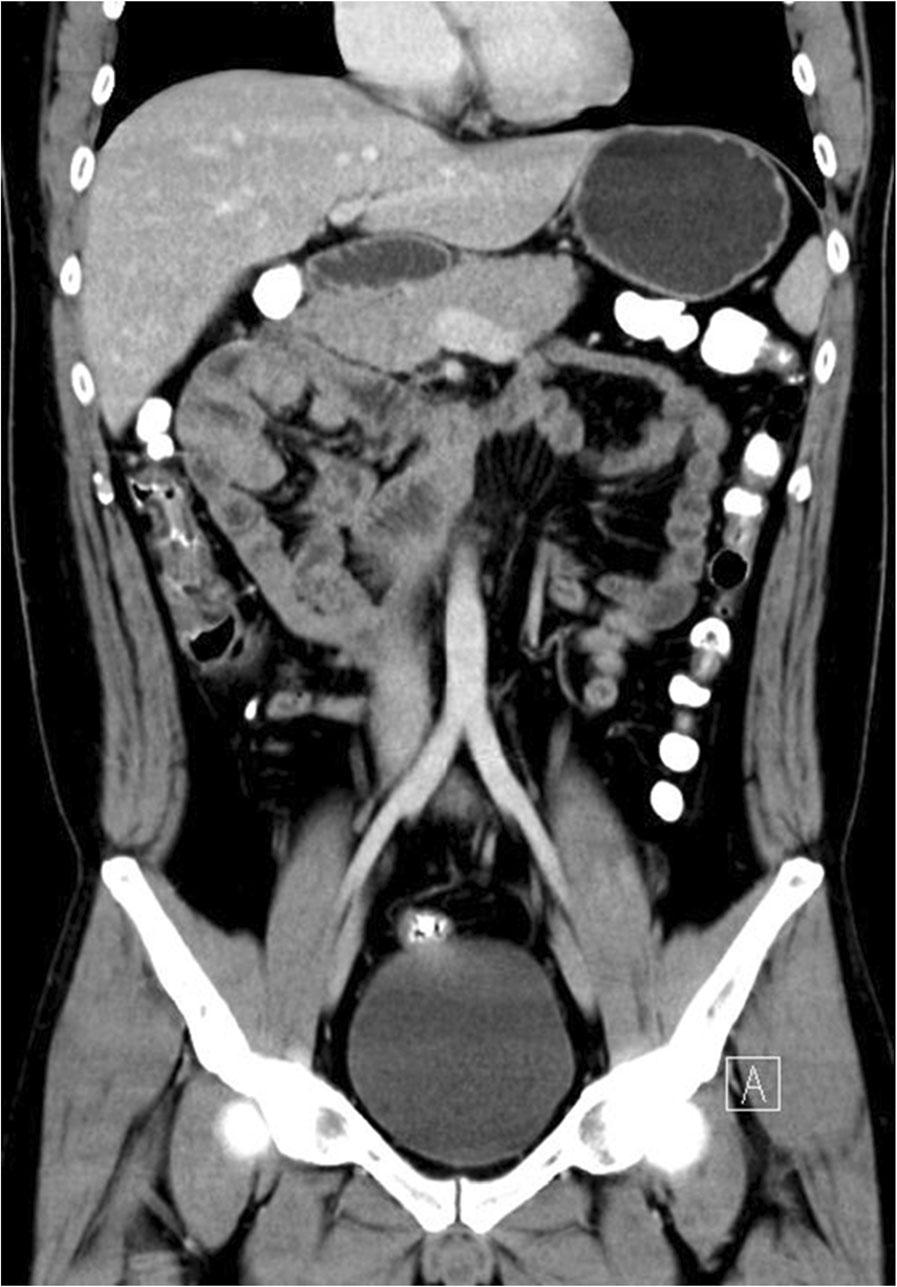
Coronary view of CT image showing the cluster of small intestine and its mesentery trapped within the right side of mesocolon
